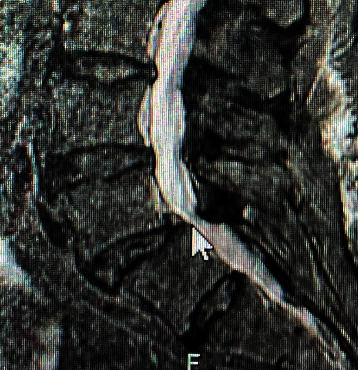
MRI lumbar spine with contrast showing an intradural and enhancing mass in the L5–S1 disc space causing severe stenosis within the intradural space. This object is an intradural enhancing mass causing severe stenosis within the intradural space.
